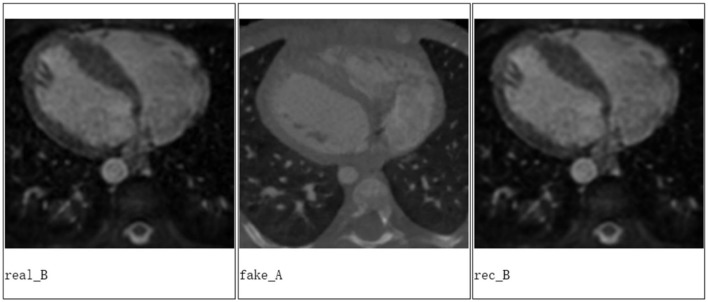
The experiment results from Cycle-GAN, where real B is the real MRI image, fake A is the generated CT image based on the real MRI image, and rec B is the reconstructed MRI image based on the generated CT image of heart for a patient.
